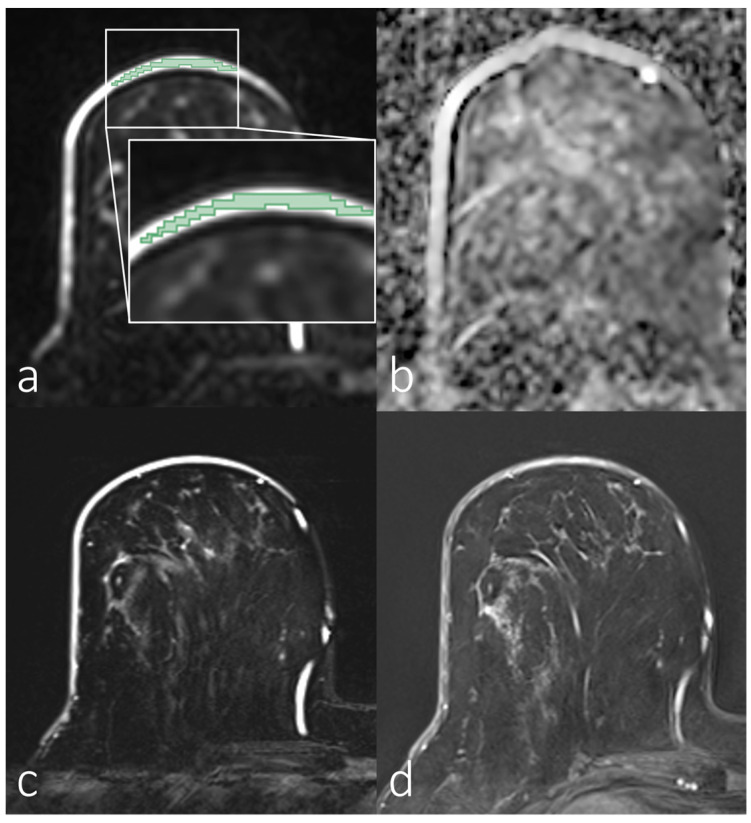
Breast magnetic resonance imaging of a woman with benign skin inflammation (mastitis). (a) Manual segmentation overlaid on the diffusion-weighted image (b = 800 s/mm^2^), with segmentation highlighted in green; (b) apparent diffusion coefficient map; (c) T_2_-weighted image with fat saturation; (d) T_1_-weighted subtraction image after contrast administration.
